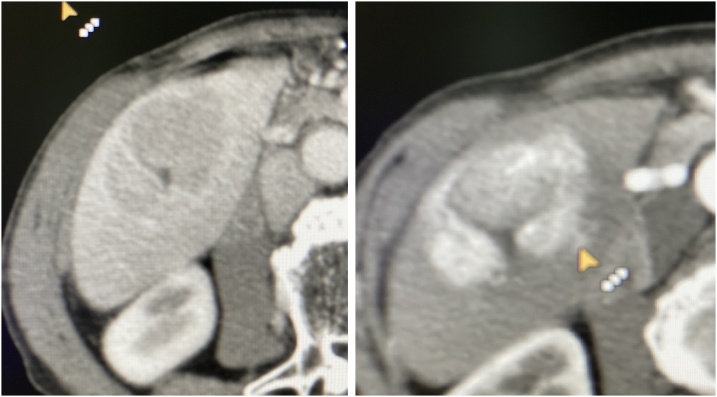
Contrast enhanced CT-scan of the abdomen showing liver tumor in segment VI with peripheral enhancement and rapid washout.
